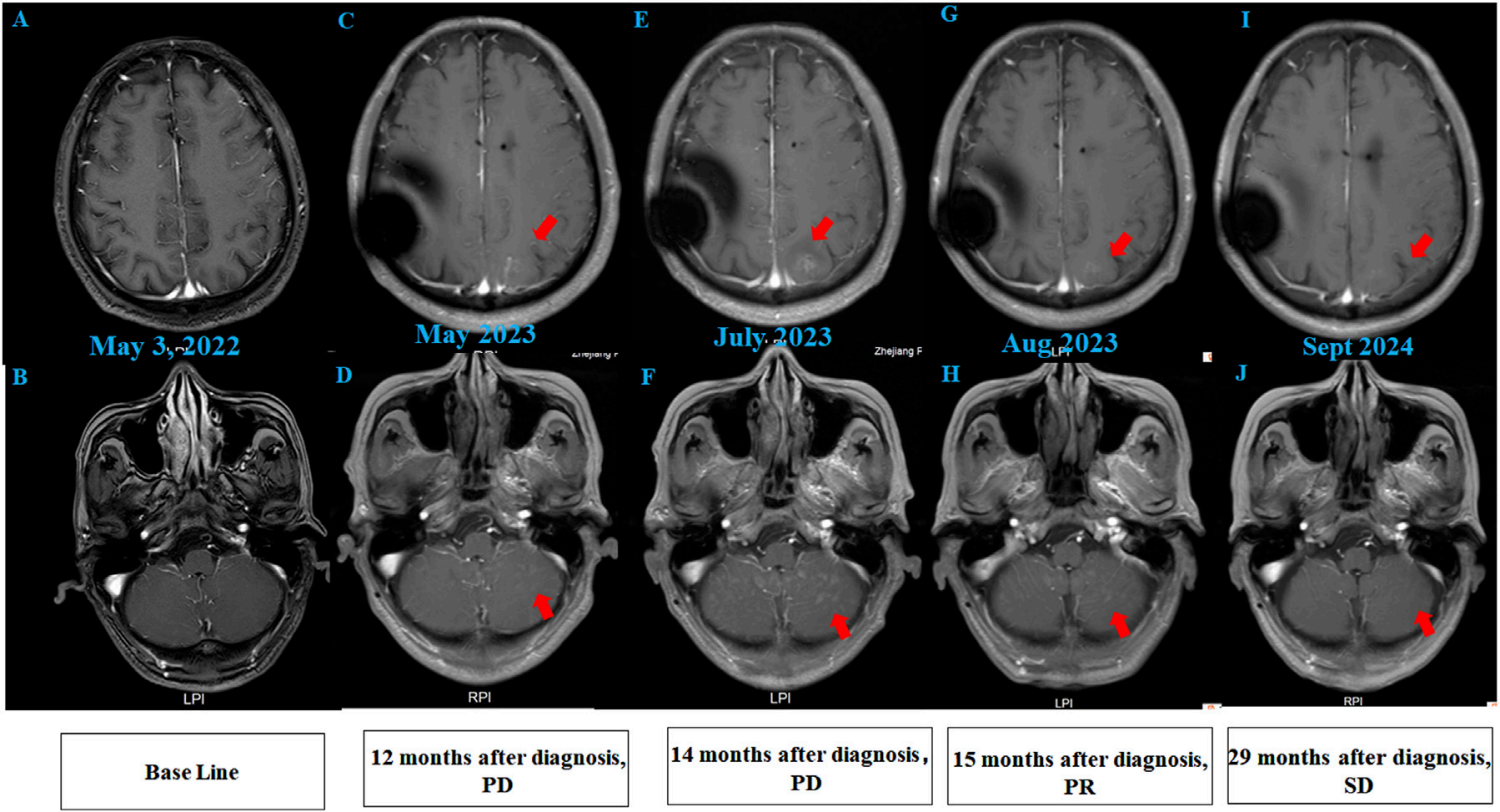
Brain MRI findings of the patient. (A,B) Initial brain MRI demonstrated T2/FLAIR hyperintensities in the white matter (Fazekas grade 1) and age-related cerebral atrophy. (C,D) MRI at 12 months post-diagnosis (after 12 cycles of intrathecal pemetrexed) revealed new abnormal signal foci in the left frontal lobe, bilateral parietal-occipital lobes, and cerebellum, indicating progressive disease (PD). (E,F) Post-whole brain radiotherapy, MRI showed increased abnormal signals compared to prior imaging. (G,H) After 2 cycles of intrathecal thiotepa, MRI demonstrated slight regression of metastatic tumors, consistent with partial response (PR). (I,J) Follow-up MRI revealed stable disease (SD), with no further progression of lesions.
